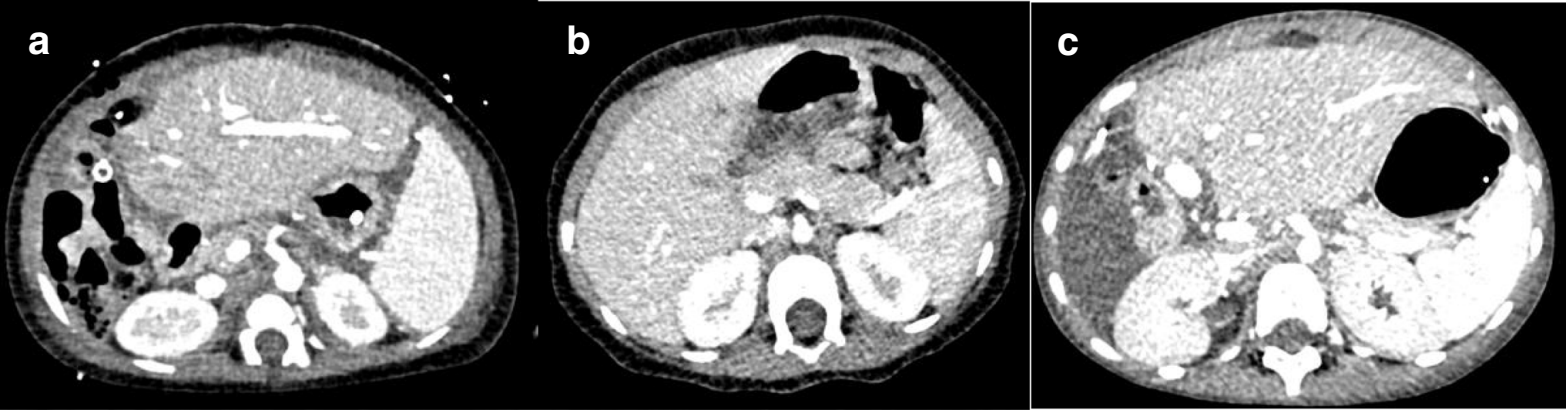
Examples of the clinical implementation of the pediatric abdomen protocol. Axial computed tomography slices in the portal venous phase after intravenous contrast demonstrate the appearances of contrast and noise of the protocol at different ages. Protocol: 70 kV Quantum mode at 53 keV virtual monoenergetic image, IQ level 120, body reconstruction kernel 40, quantum iterative reconstruction 2, 1.0-mm slice thickness. a Six-month-old girl after liver transplantation. b Two-year-old girl prior to liver transplantation. c Six-year-old boy after liver transplantation
